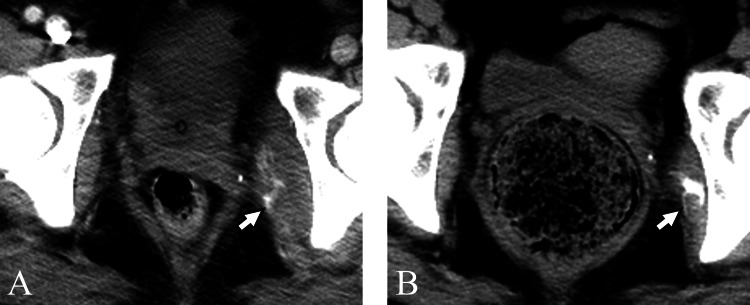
Case six A 35-year-old woman with myositis ossificans in the left short external rotator muscles. (A) CT obtained on the 36th day of hospitalization showed swelling in the left muscles (arrow). Manual muscle testing score in the left lower limb was 1. Acute pancreatitis was present as the cause of fever. (B) On the 53rd day, CT displayed calcification within the left muscles (arrow). (C) On the 136th day, CT demonstrated ossification and a decrease in swelling (arrow).
